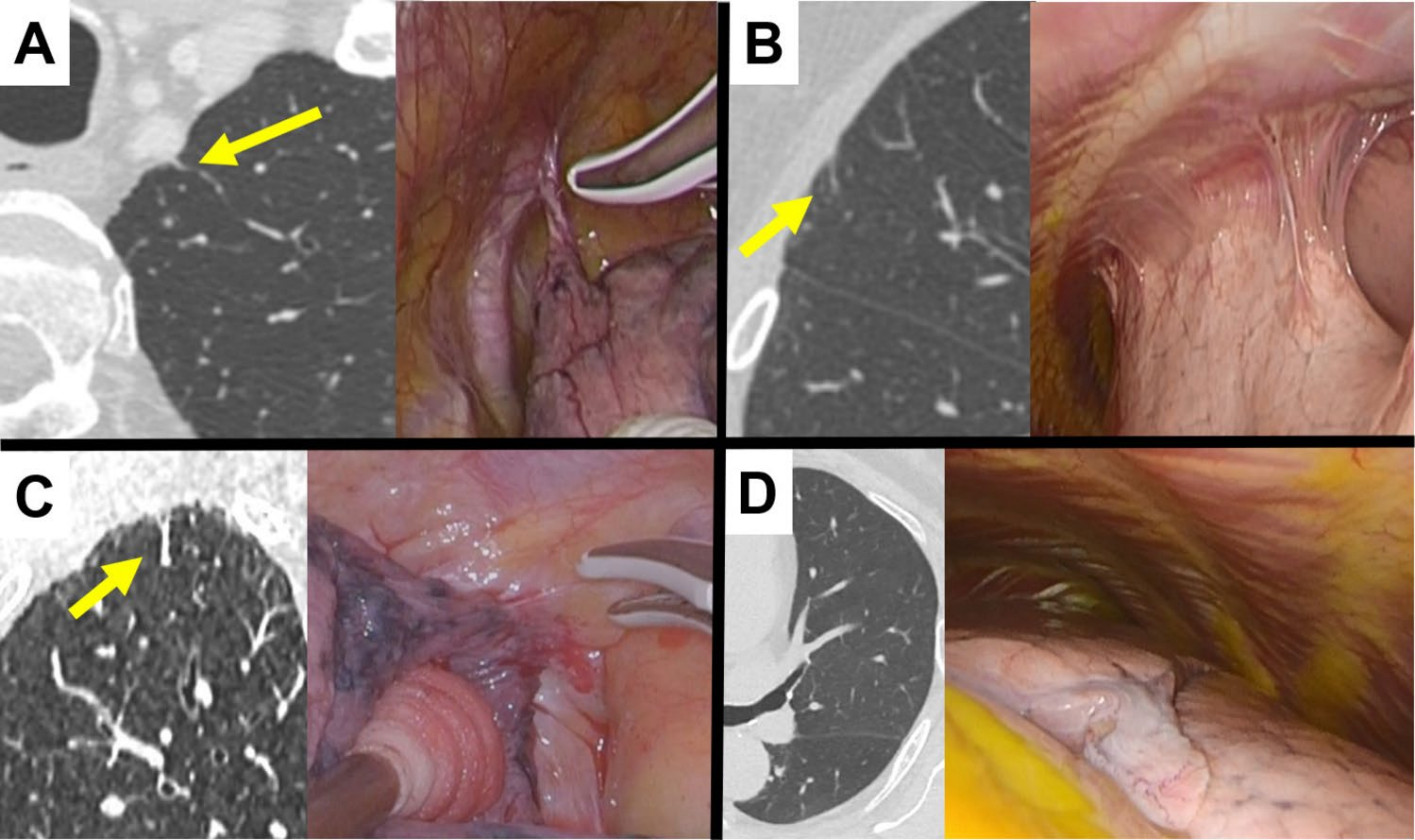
A–C Micro-vessels beneath the pleural surface (MVBP) were detected on preoperative computed tomography (CT), suggestive of the presence of pleural adhesions in these patients. At the time of surgery, pleural adhesions were confirmed to be present. D MVBP were undetectable on preoperative CT and the absence of pleural adhesions was suspected in this patient. At the time of surgery, pleural adhesions were confirmed to be absent. *MVBP* micro-vessels beneath the pleural surface
